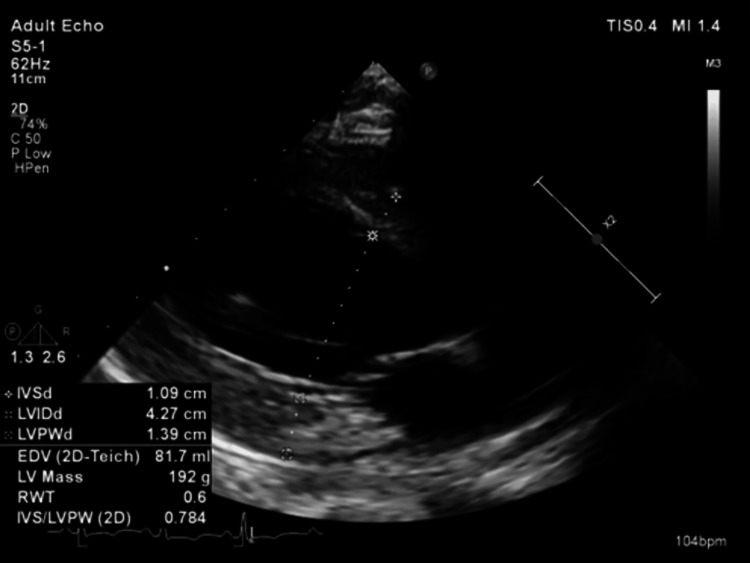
Parasternal long-axis view revealing concentric left ventricular hypertrophy with normal cavity size
